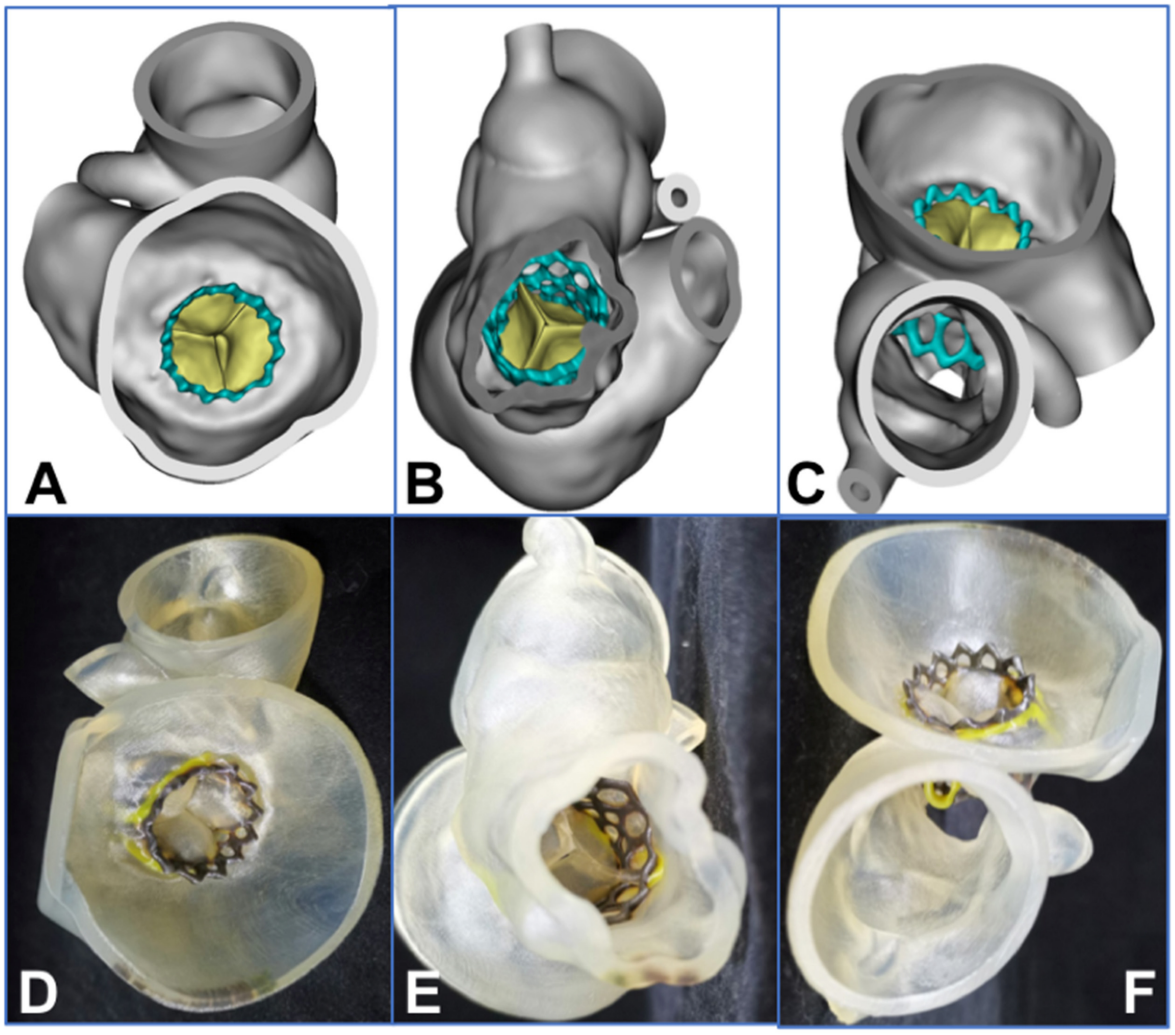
Assessment of 3-dimensional (3D) reconstruction is completed by using post-procedural computed tomographic data (e.g., data for patient 2). (A–C) 3D reconstruction from the plane of the left atrium, the left ventricle, and the ascending aorta, respectively. (D–F) The 3D printed model from the plane of the left atrium, the left ventricle, and the ascending aorta, respectively. The yellow area represents the frame of the degenerated valve, and the black area is the frame of the balloon-expandable valve.
